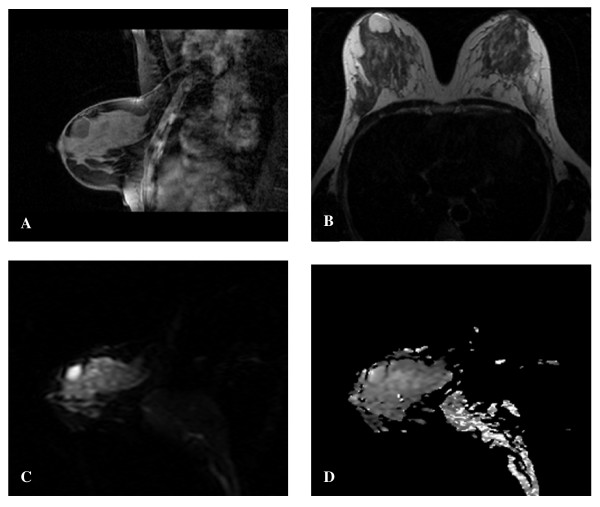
36-year-old woman with fibroadenoma in right breast. A: WATS T1-weighted sagittal scan showed a regular round slightly hypointense lesion. B: T2-weighted transverse scan showed a round and smooth hyperintense lesion in the right breast. C: EPI-DWI sagittal scan showed a hyperintense lesion in DWI. D: The isointense lesion was in ADC map (b = 1000) and the ADC was 2.44 ± 0.362×10^-3^mm^2^/s.
